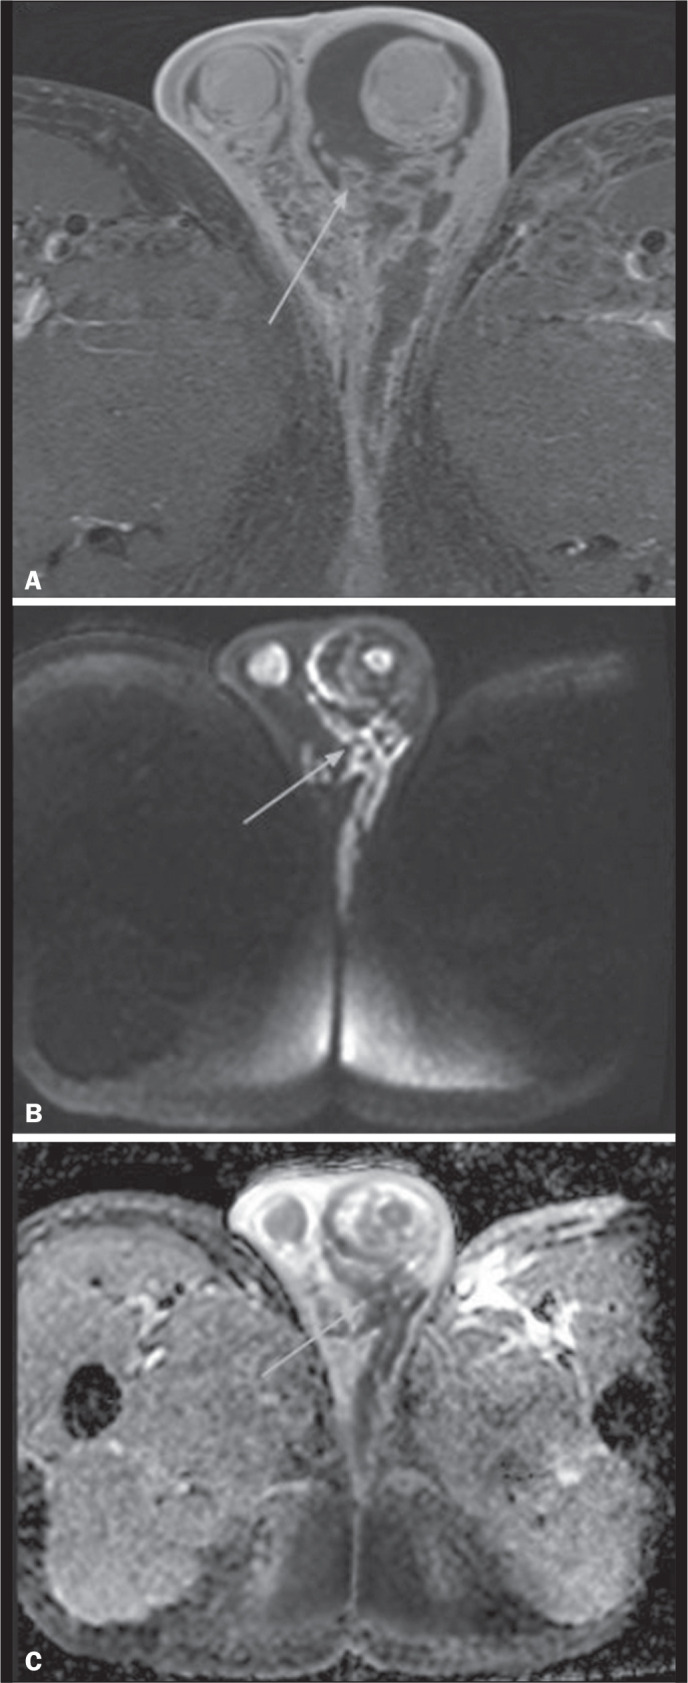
Abscesses and pyoceles. Contrast-enhanced axial T1-weighted sequence
